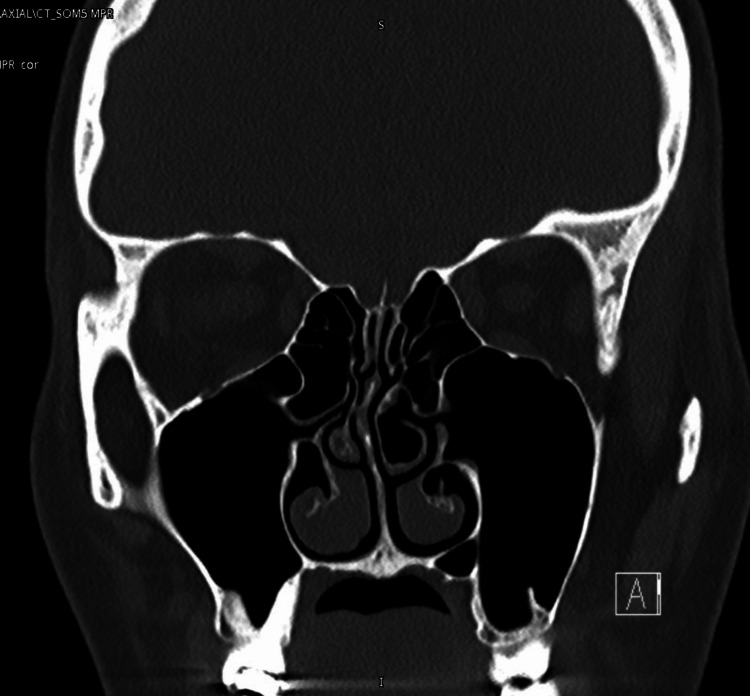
Preoperative CT scan (coronal view)
